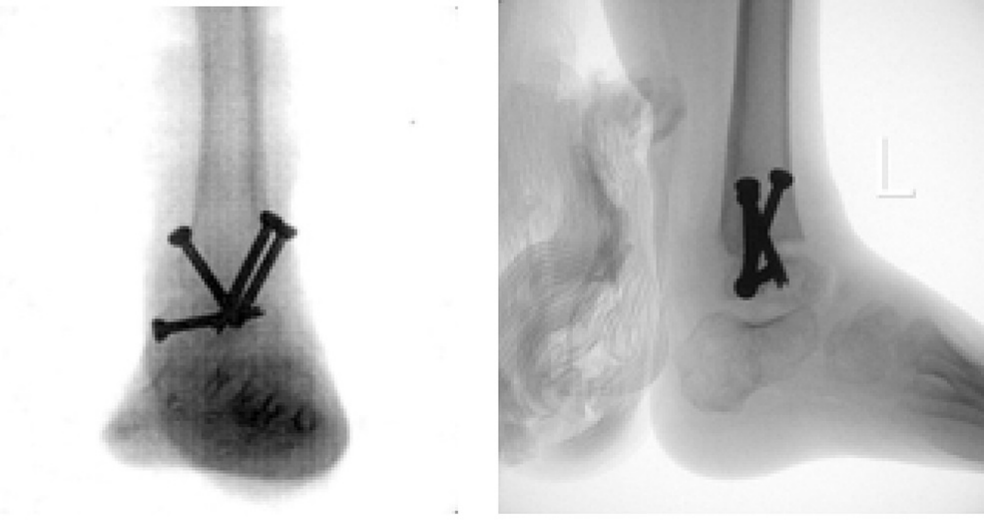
Intraoperative fluoroscopy Posteroanterior (Panel A) and lateral (Panel B) intraoperative radiograph demonstrating a left tibiotalar arthrodesis with internal fixation and resolved superolateral subluxation.
